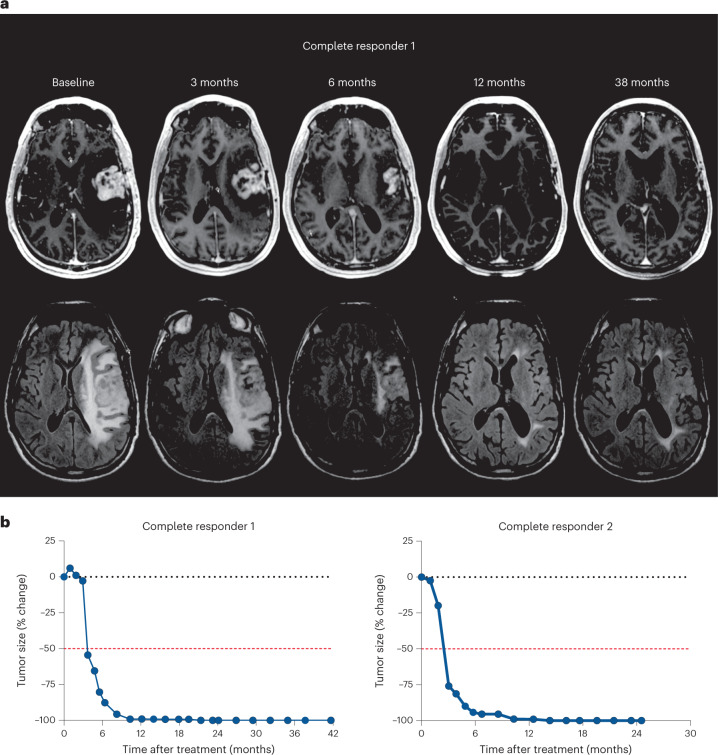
Complete responses to DNX-2401 and pembrolizumab. a, Axial T1-weighted MR (top row) and FLAIR images (bottom row) obtained at baseline, 3 months, 6 months, 12 months and 38 months after infusion of DNX-2401 for one complete responder. b, The change of tumor size over time in each patient with a complete response. Dotted black line represents no change relative to baseline. Dashed red line represents the threshold for response according to the mRANO criteria. Both patients showed response to treatment at 3 months after DNX-2401 infusion, with complete response by 15–18 months.
